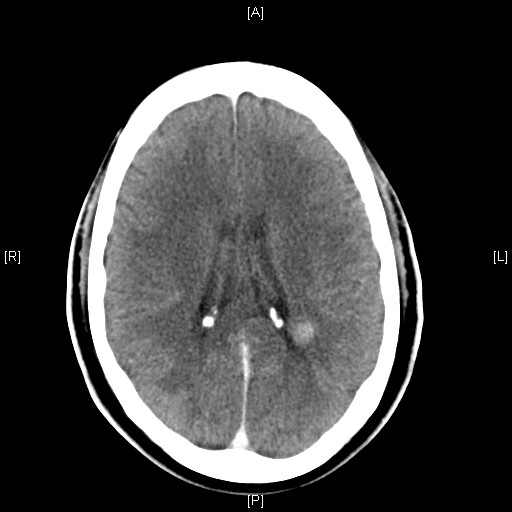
Brain computed tomography scan of the patient on presentation.
